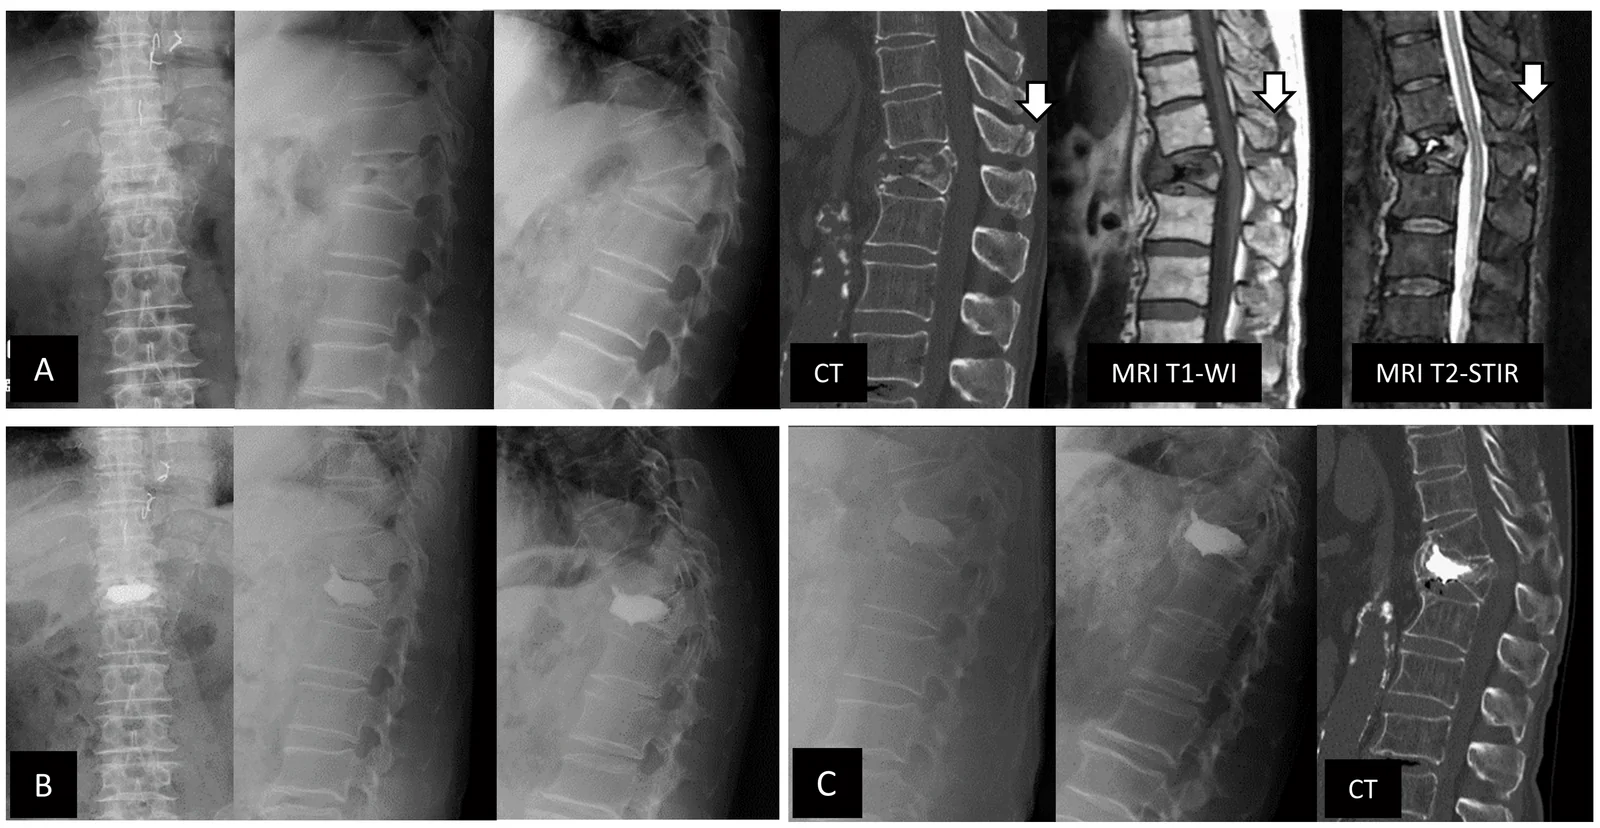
Preoperative and postoperative images of an 80-year-old male with a T12 vertebral fracture A) Preoperative images: from the left - radiographs (anteroposterior, supine lateral, seated lateral), computed tomography (CT), magnetic resonance imaging (MRI) (T1-WI, T2-STIR). White arrows indicate the spinous process fracture line; B) One week postoperative: from the left - radiographs (anteroposterior, supine lateral, seated lateral); C) Two years postoperative: from the left - radiographs (supine lateral, seated lateral), CT (sagittal view).
